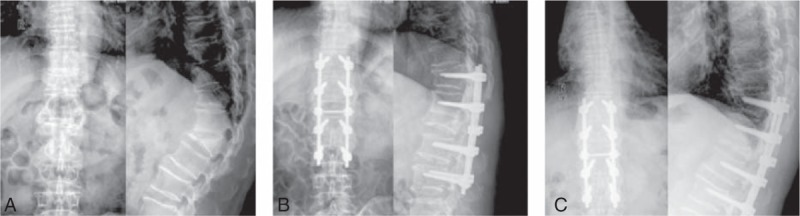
The comparison of the preoperative, intraoperative, and postoperational X-ray images in patients with traumatic kyphosis after posterior spinal V osteotomy. Note: (A) The front and lateral X-ray image before posterior spinal V osteotomy; (B) the front and lateral X-ray image of correction after posterior spinal V osteotomy; (C) X-ray showed that the fixation was firm and absence from looseness 6 months after operation.
